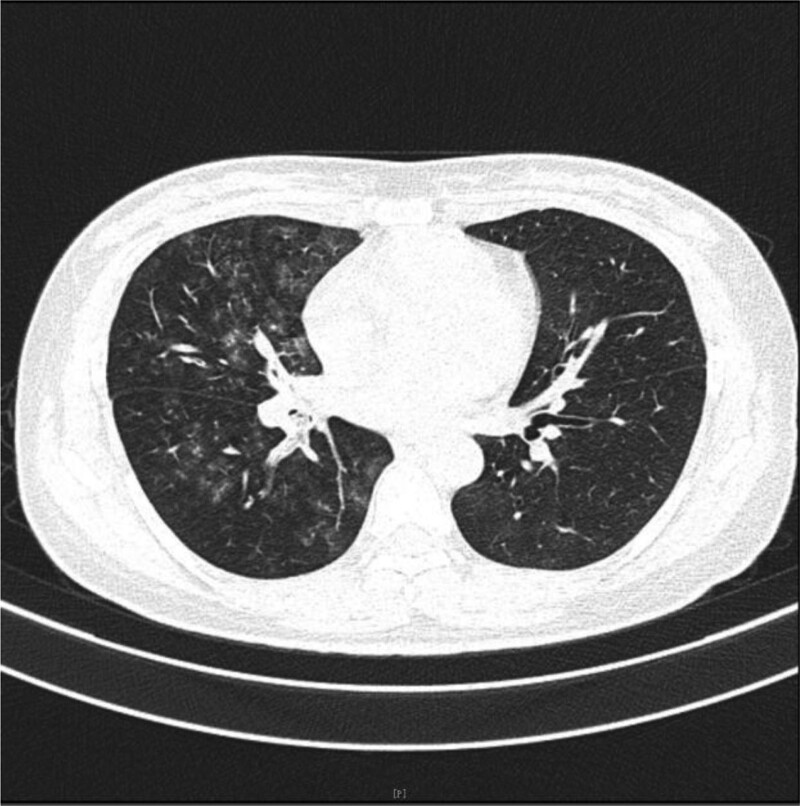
On May 17th, emergency chest CT showed multiple patchy and nodular ground-glass opacities in both lungs, with partial consolidation. The right lung was predominantly affected, showing some changes resembling the “paving stone” sign. Increased density opacities were observed within the right main bronchus, as well as the middle and lower lobes of the right lung. Bilateral pneumonia was considered, accompanied by alveolar hemorrhage, and multiple bronchial mucus plugs in the right main bronchus and bronchi of the middle and lower lobes.
